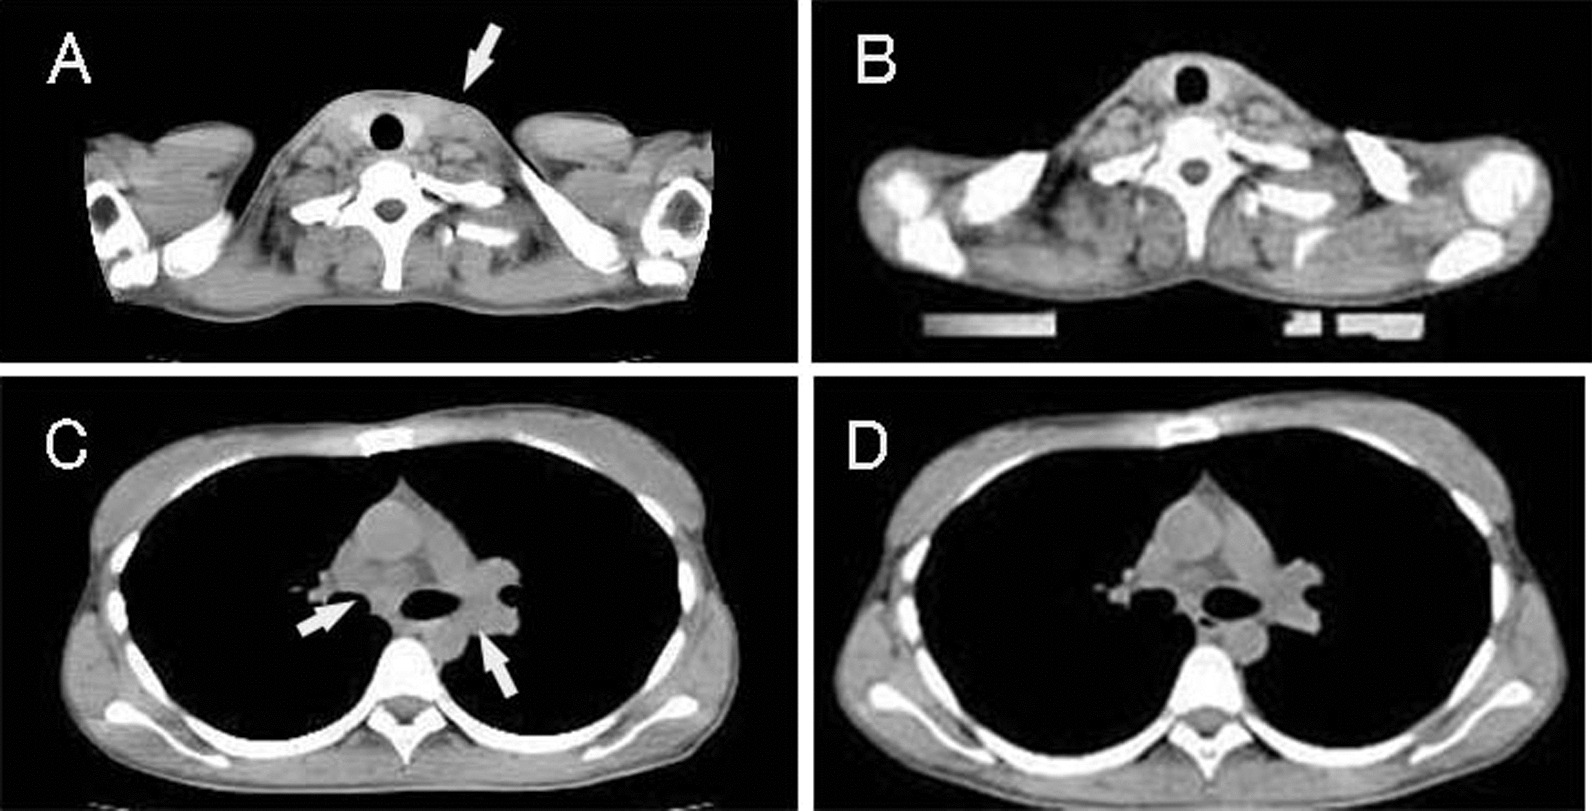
Computed tomography images. A, B Supraclavicular area and C, D mediastinal area. A, C Before and B, D after chemoradiotherapy. The arrow in A indicates enlarged left supraclavicular lymph nodes, and the arrows in C indicate enlarged bilateral hilar lymph nodes
